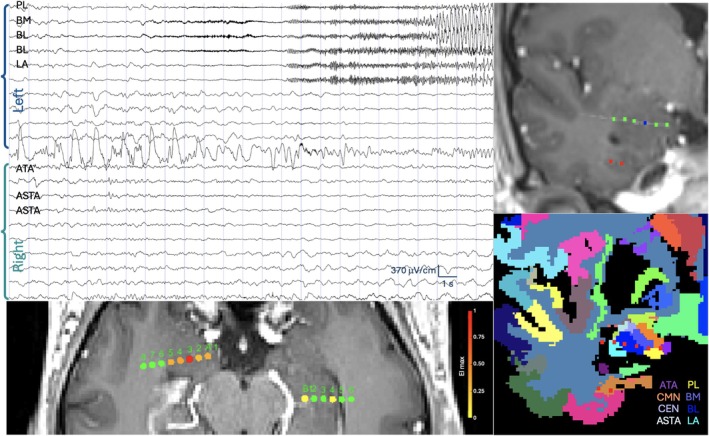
Example of the amygdala involvement in a left medial temporal seizure. A 36‐year‐old right‐handed patient with magnetic resonance imaging (MRI) ‐negative, drug‐resistant focal epilepsy underwent stereoelectroencephalography (SEEG) as part of routine presurgical work‐up, with the main hypothesis of the left temporal mesial‐basal epileptogenic zone (EZ). A left‐predominant bilateral implantation was performed (15 left and 2 right electrodes) to delineate the EZ, assess its relationships with functional areas, and evaluate the contribution of contralateral mesial temporal structures to the EZ network extent and functional prognosis. Right: automated visualization and labeling of electrodes in patient's anatomy using the Gardel software. Top right. Coronal view of the patient's 3D T1 MRI (displayed in neurological convention) showing localization of the A' electrode contacts within the patient's left amygdala (selected contact A'3 (left BL) is lighted in blue, other A' contacts in green). Bottom right. A' electrode contacts (red dotes) overlayed onto the amygdala nuclei parcellation (Tyszka and Pauli atlas merged with the virtual epileptic patient [VEP] atlas) projected in the patient's MRI space. Abbreviations: ASTA = amygdalo‐striatal transition area; ATA = periamygdaloid cortex; BL = basolateral nucleus; BM = accessory basal nucleus; CEN = central nucleus; CMN = corticomedial nucleus; LA = lateral nucleus; PL = paralaminar nucleus. Top left. The 25‐seconds ictal SEEG signal dataset focusing on the contacts within the left and the right amygdala highlighting 6 sampled amygdala nuclei (left PL, BM, BL, and LA; right ATA and ASTA). Bottom left. Axial view of the patient's 3D T1 brain MRI (displayed in neurological convention using 3D‐Viewer) highlighting the A' electrode targeting the left amygdala, and the B electrode targeting the right hippocampus, with graphical representation of normalized Epileptogenicity index (EI) ^4^ values (color scale from green (EI = 0) to red (EI = 1) for intracranial contacts with the lowest to the highest epileptogenicity). Following epileptogenicity values were obtained across the sampled amygdala nuclei in this patient: EI values: PL, 0.32; BM, 0.27; BL, 1; LA, 0.31; ATA, 0; ASTA, 0.002; Connectivity Epileptogenicity Index (cEI) values: PL,0.33; BM, 0.35; BL, 1; LA, 0.35; ATA, 0; ASTA, 0.002. [Color figure can be viewed at www.annalsofneurology.org]
